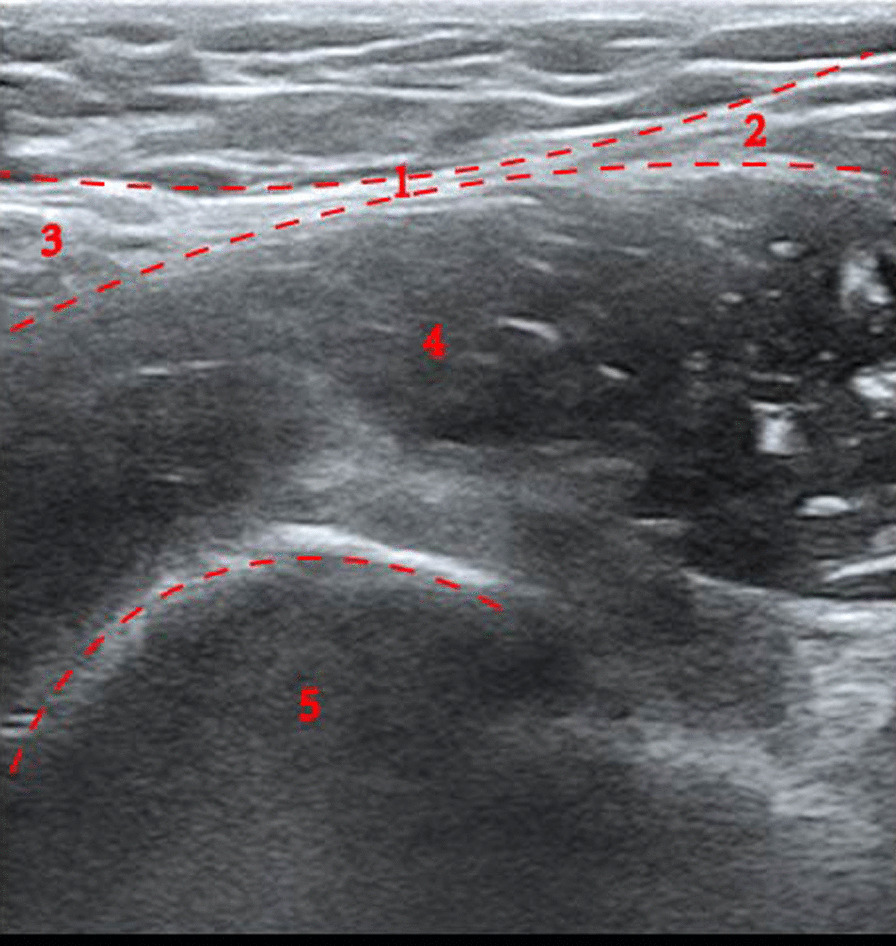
Fascia iliaca compartment block: bow-tie in the iliac fascia region on ultrasound. 1. Bow tie sign 2. Sartorius muscle 3. Internal oblique muscle 4. Iliac muscle 5. Anterior superior iliac spine
